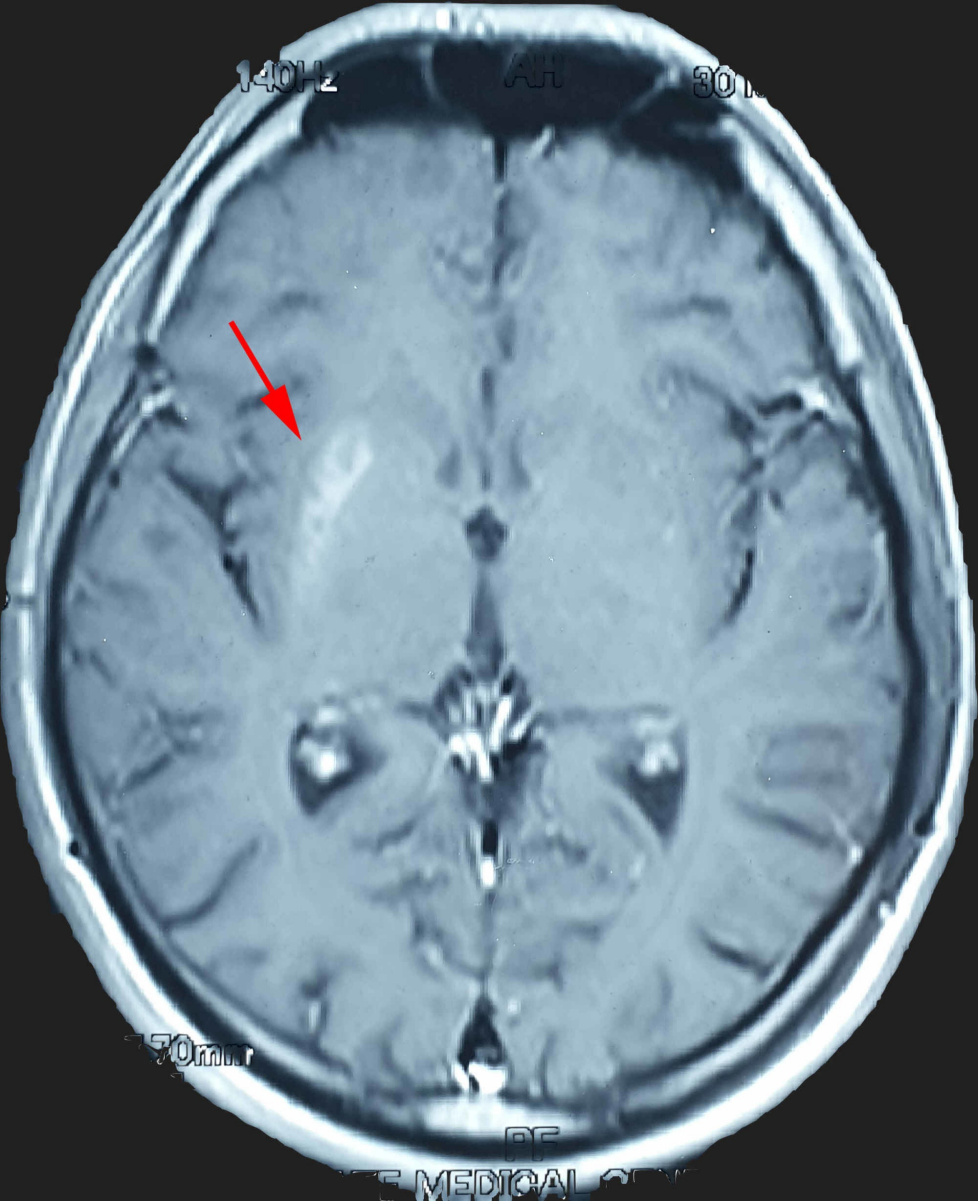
T1 Weighted MRI brain image. Arrow points toward right-sided basal ganglia lesion.
